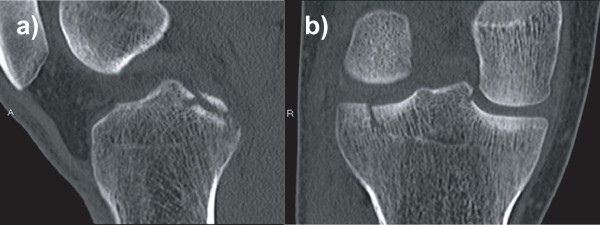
Computed tomography scans showing sagittal and coronal views of the knee: the displaced fragments of the posterior intercondylar eminence (a) and the avulsed lateral tibial condyle (b) can be observed.
